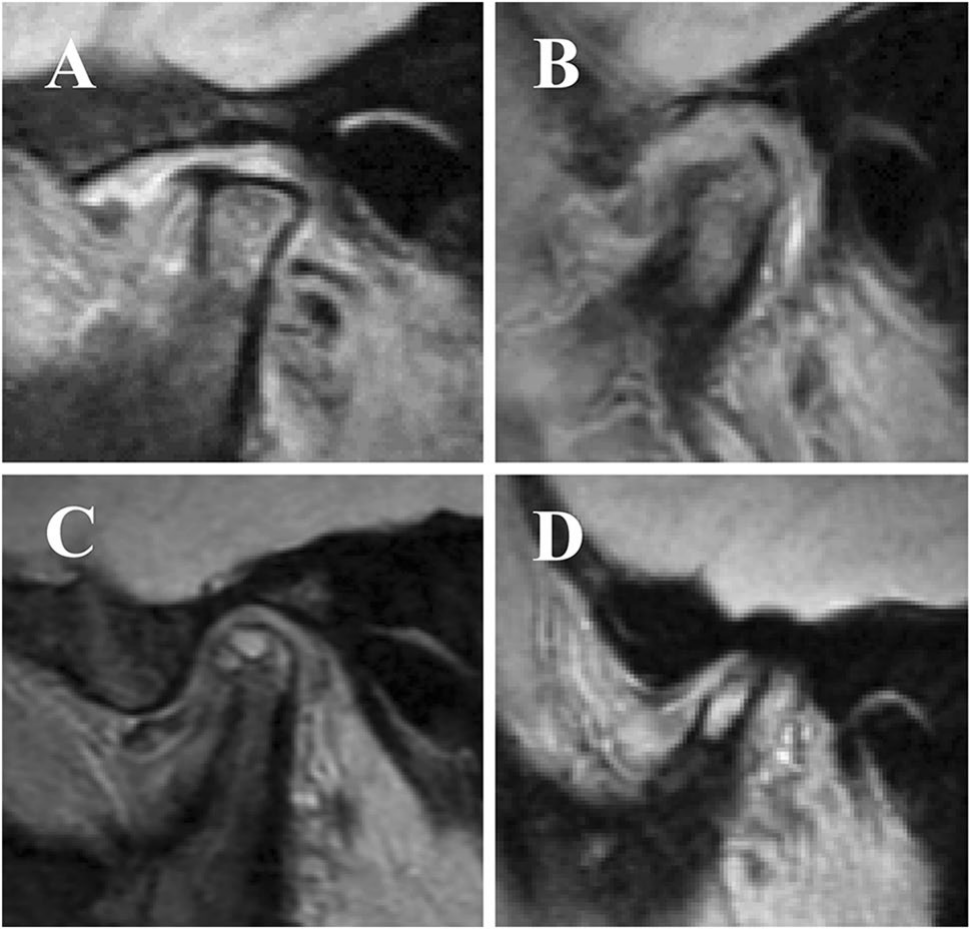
Examples of osteoarthritis on MRI. A Osteophyte. B Erosion. C Subchondral cyst. D Atrophic deformity
